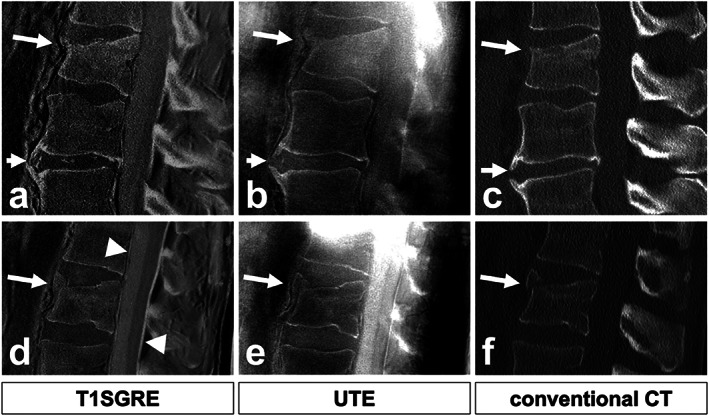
Comparison of T1SGRE‐derived CT‐like images (a, d), UTE images (b, e), and conventional CT images (c, f). In one patient (a–c), a wedge‐compression fracture of L1 with signs of an acute pathology such as a compaction zone can be depicted (upper arrows), as well as ventral and small dorsal osteophytes on level L2/3 (lower arrows). In another patient (d–f), another wedge‐compression fracture of L2 with a triangular teardrop‐like fragment can be identified (arrows). Also note the thin hyperintense line running longitudinally along the posterior walls of vertebral bodies representing the posterior longitudinal ligament as well as the thicker hyperintense line posterior to the dural sac representing the ligamenta flava (arrowheads; d), which are not depicted on CT (f), and must not be misinterpreted as ligament calcifications. Figure reproduced without modification from reference 36 under the Creative Commons Attribution 4.0 International License (http://creativecommons.org/licenses/by/4.0/). T1SGRE = T1 radiofrequency spoiled gradient‐echo; UTE = ultrashort echo time; CT = computed tomography.
